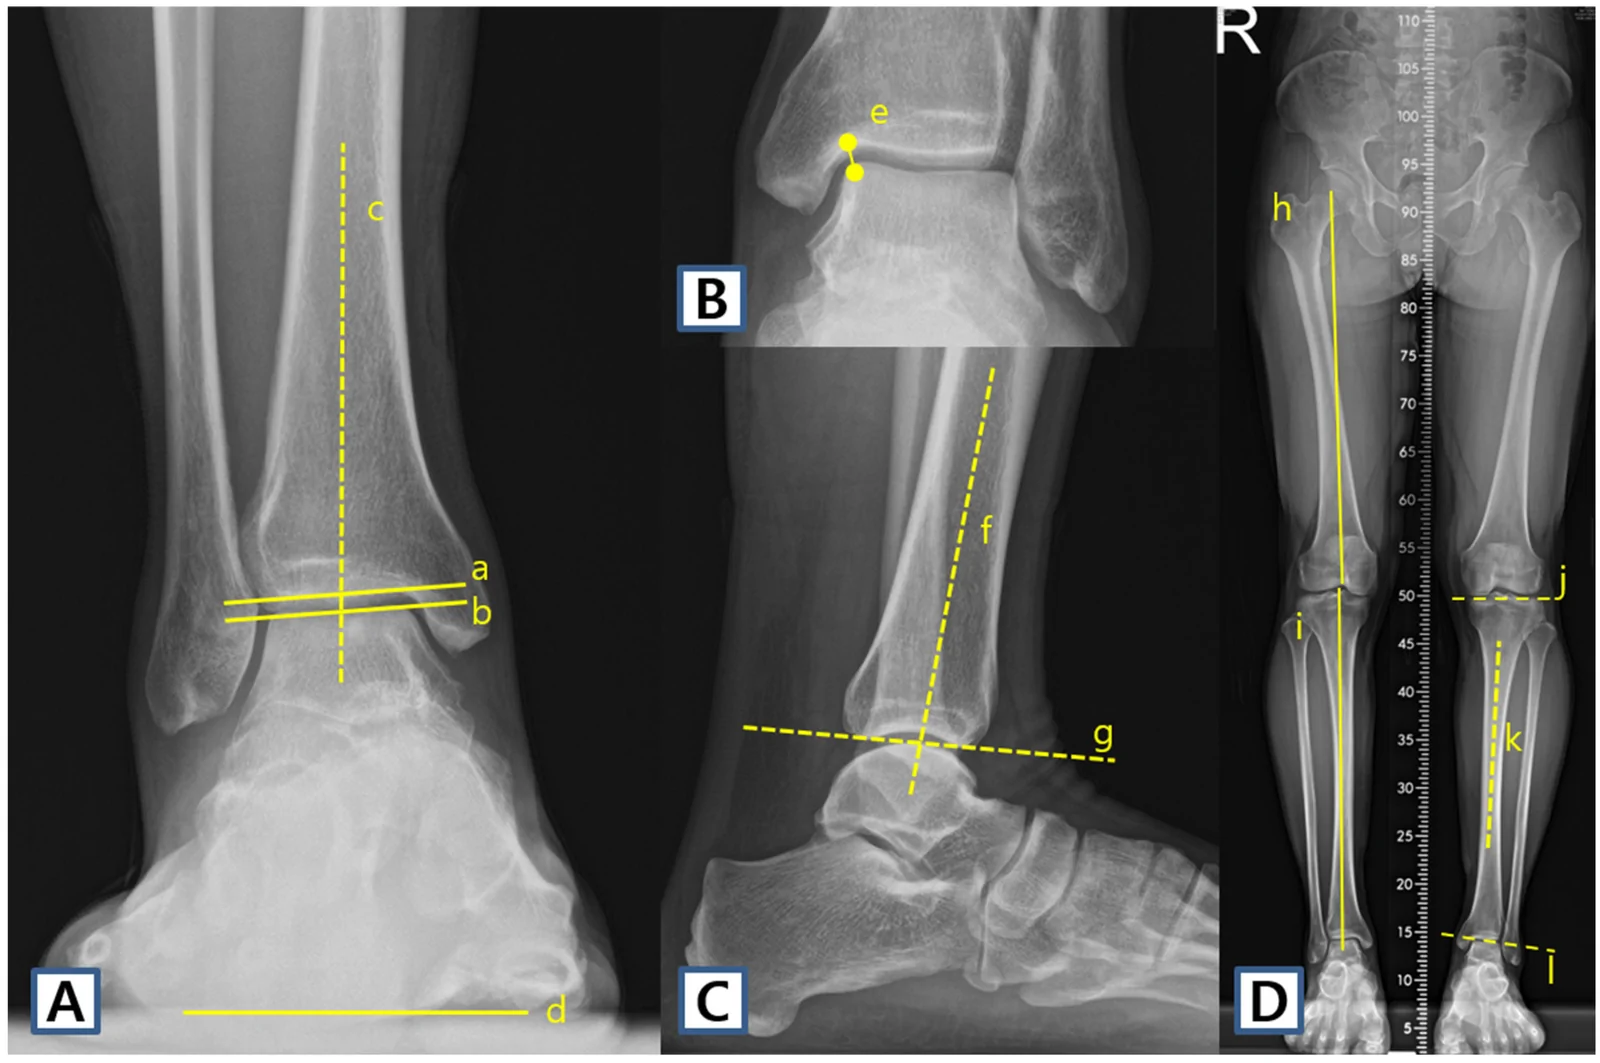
Radiologic parameters in lower extremity scanogram and standing Ankle X-ray: (A) Talar tilt angle (a and b), tibial anterior surface angle (a and c), tibial plafond inclination (a and d), talar inclination (b and d). (B) The medial clear space (e) was defined as the shortest distance between the lateral border of the medial malleolus and the medial border of the talus at the level of the ankle mortise. (C) Tibial lateral surface angle (f and g) was evaluated in the ankle lateral radiograph. (D) Hip-knee-ankle angle (h and i), medial proximal tibial angle (j and k), knee-tibial plafond angle (j and l) was measured on full-length standing lower extremity scanograms. Leg length discrepancy was measured as the difference in the distance from the center of the femoral head (hip center) to the center of the talar dome between the two lower extremities.
